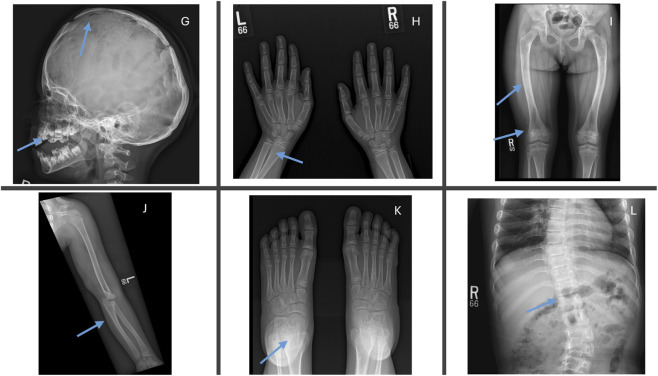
Follow-up skeletal survey at 6 years of age post-asfotase alfa therapy (Case 12). (G) Skull radiograph showing persistent lacunar appearance, mild midface hypoplasia, and abnormal dentition. (H) Hand radiograph demonstrating improved mineralization but persistent cortical thinning and mild negative ulnar variance. (I) Lower limb radiograph showing persistent bowing of the femurs and improvement in metaphyseal morphology. (J) Upper limb radiograph depicting mild negative ulnar variance bilaterally. (K) Foot radiograph with evidence of broadening and widening of the tarsal and metatarsal bones. (L) Spinal radiograph revealing an S-shaped thoracolumbar scoliosis with focal kyphosis at the thoracolumbar junction.
